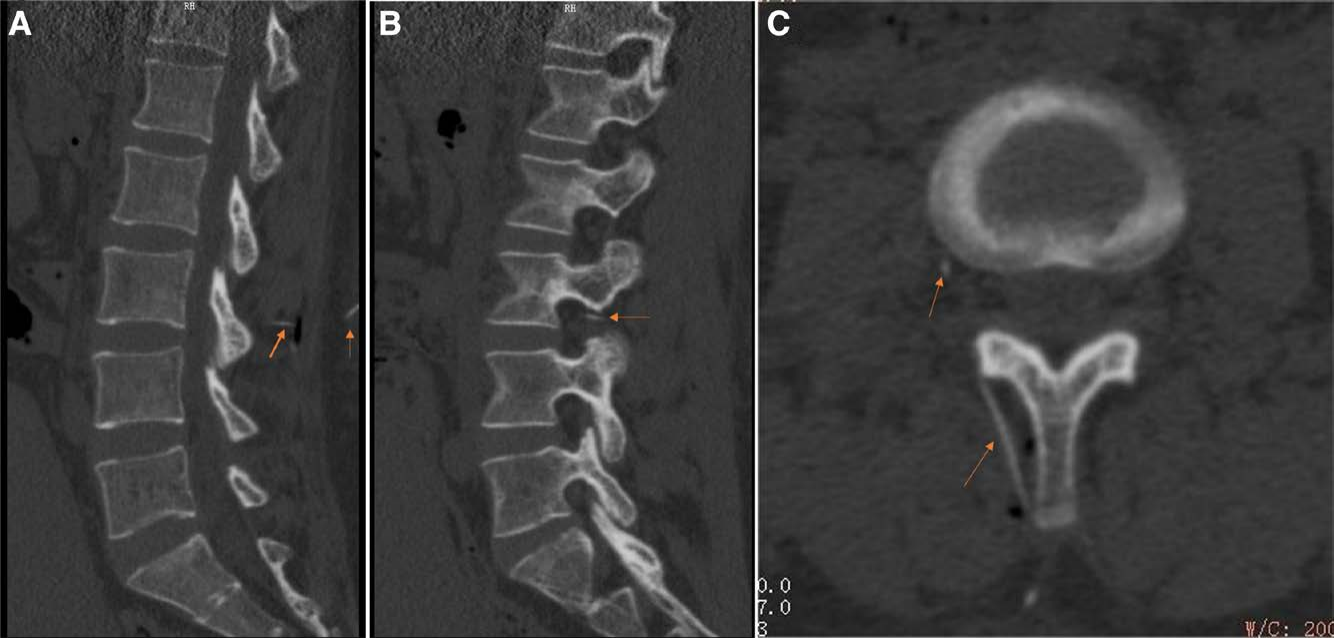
CT images. (A) Sagittal view of the CT spine demonstrating epidural catheter (arrow) that extends in via the midpoint of the L2–3 interspinous space at the vertebral level of L3. (B) Sagittal view of the CT spine showing the epidural catheter (arrow), which after its deviation from the midline is located at the right side close to the L3–4 intervertebral foramen. (C) Transverse view of the CT spine showing the epidural catheter (arrow) continues to course significantly off midline to the right posterior lower aspect of the L3 vertebral body.
